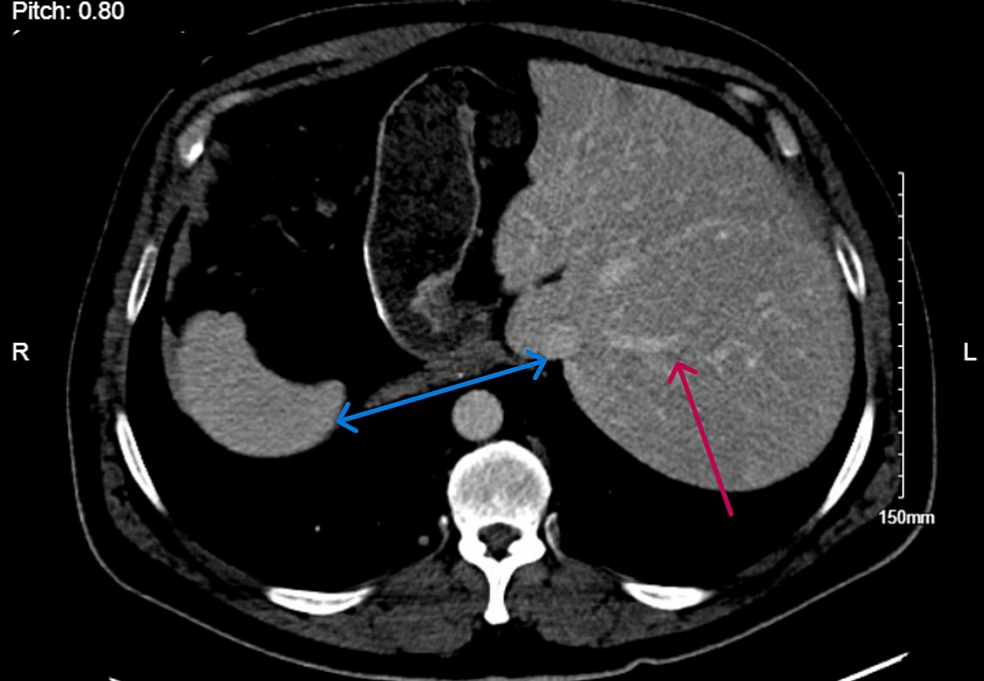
CT abdomen with contrast showing situs inversus (blue double-headed arrow), fatty liver and no focal hepatic lesion (red arrow)
